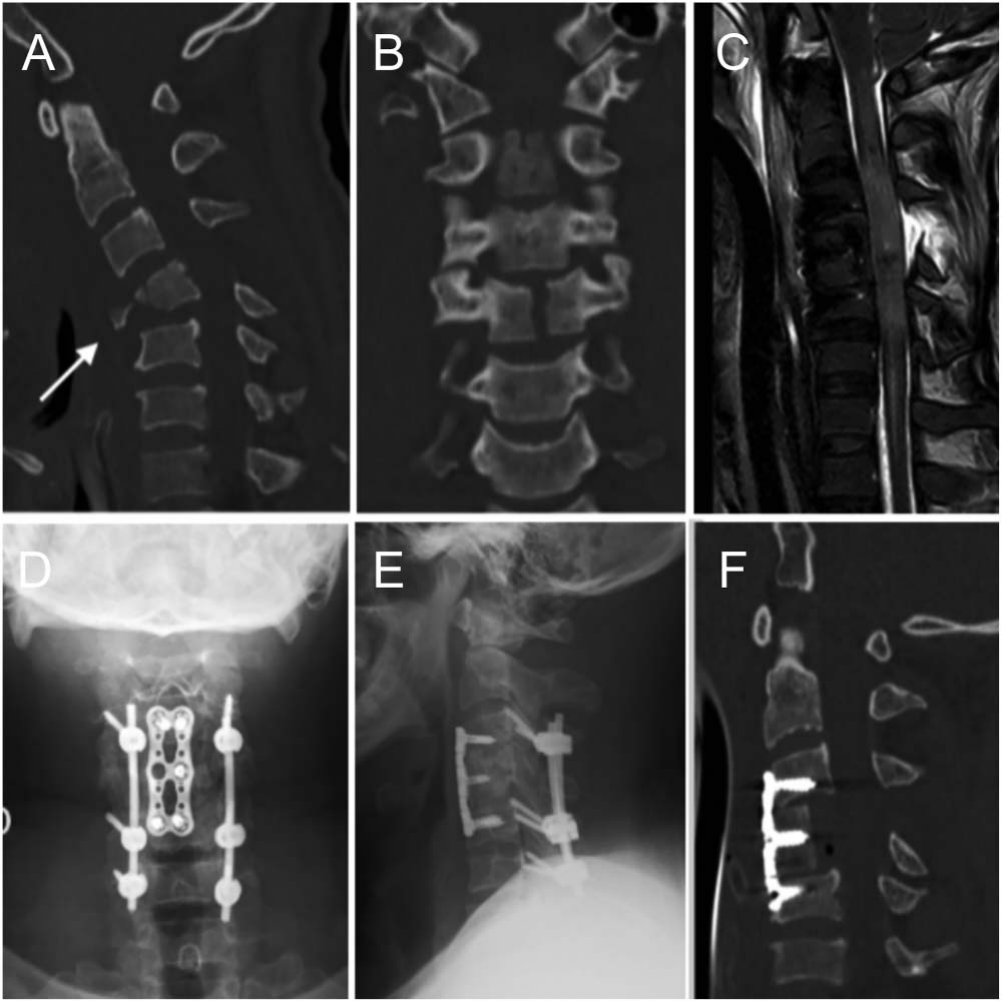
C3 teardrop fracture. (A and B) Sagittal and coronal CT scans of the spine. C: MRI showing signal alterations. (D and E) Postoperative X-rays after combined anterior and posterior fixation, plus autograft. (F) Postoperative CT scan.
